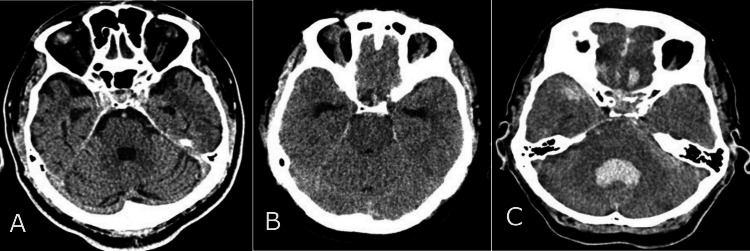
Basal cisterns (BC) CT findings CT normal findings of BC (A); compressed BC (B); absent BC (C).
